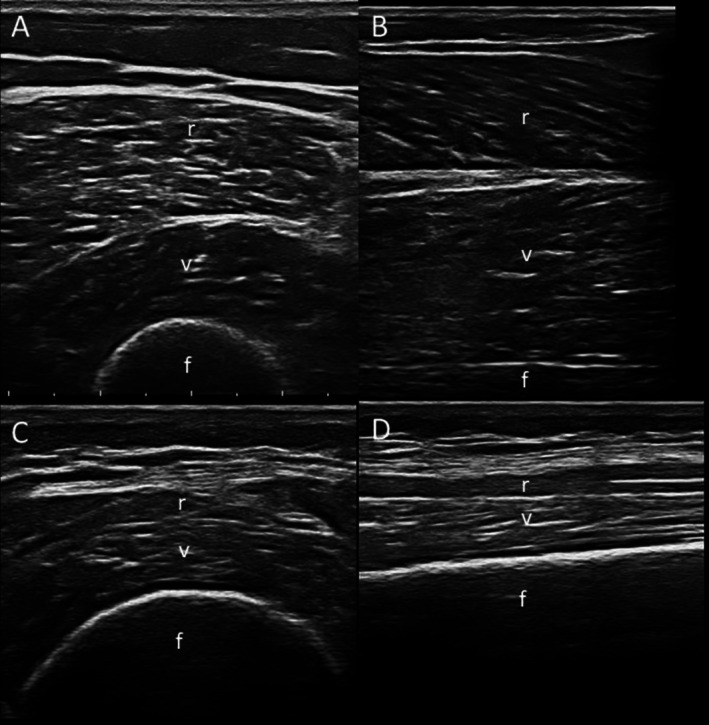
Sarcopenia assessment using ultrasound (US). Images acquired with a linear probe (3–13 MHz). Comparison between a healthy subject (40‐year‐old woman who regularly engages in sports activities) and a sarcopenic subject (88‐year‐old woman with rheumatoid arthritis). Transverse (A, C) and longitudinal (B, D) US images of the anterior thigh compartment muscles (rectus femoris, vastus intermedius). Images (A) and (B) correspond to the healthy subject; images C and D correspond to the sarcopenic subject. (C) and (D) images show a reduced thickness of muscles bellies, and an increased muscles echogenicity compared to (A) and (B) (i.e., Grade III of the Heckmatt scale [i.e., marked increased muscle echo with reduced bone echo] vs. Grade I of the Heckmatt scale [i.e., normal hypoechoic muscle], respectively).f, femur; r, rectus femoris; v, vastus intermedius.
